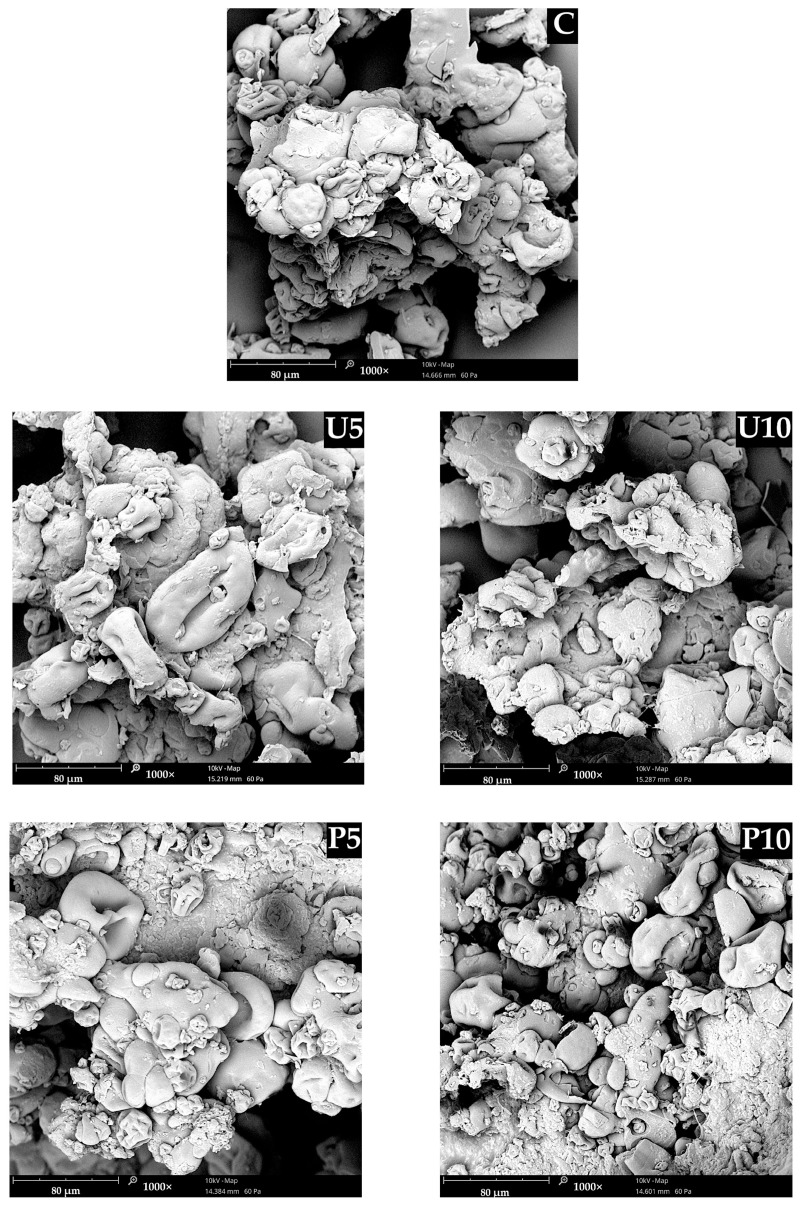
Effects of sequential thermal and non-thermal induction on the microstructure of the hydrogels. Magnification 1000×. Samples description: C—Control hydrogel induced via thermal induction; U5 and U10—Thermal induction followed by ultrasound treatment for 5 and 10 min, respectively; P5 and P10—Thermal induction followed by high hydrostatic pressure treatment for 5 and 10 min, respectively.
